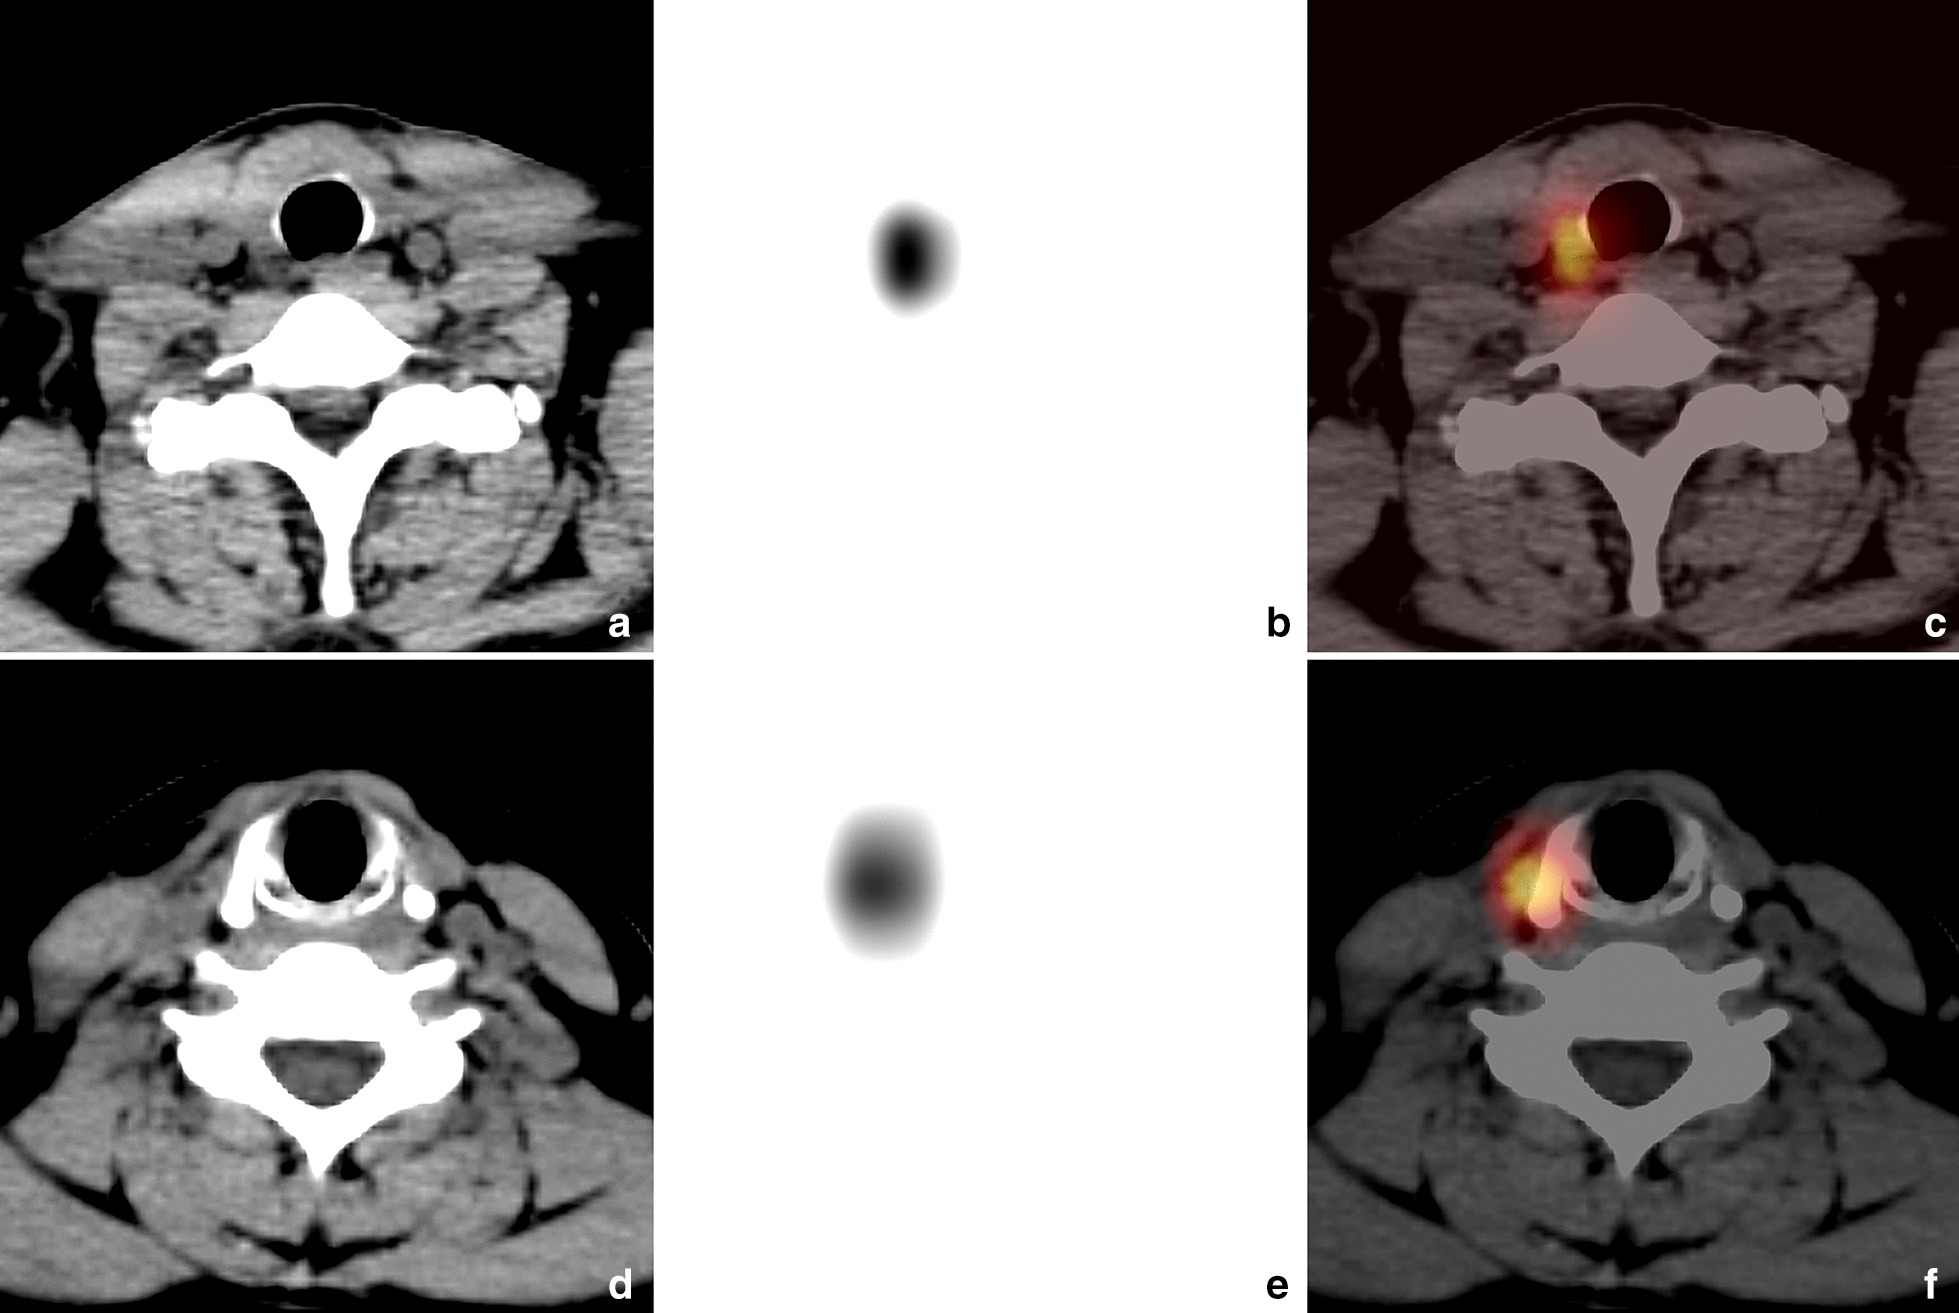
RTT lesions of type II (suspected positive CT findings). The images (a CT; b SPECT; c fusion) were obtained from a 38-year-old man with PTC, who underwent total thyroidectomy 3 months ago. SPECT/CT demonstrated a RTT lesion with high-uptake and suspected thickened soft tissue, adjacent to the right side of tracheal cartilage (region I). The images (d CT; e SPECT; f fusion) were obtained from a 31-year-old woman with PTC, who underwent total thyroidectomy 2 months ago. SPECT/CT revealed a RTT lesion with high-uptake and blurry patchy shadow, adjacent to the thyroid right lamina (region IV)
